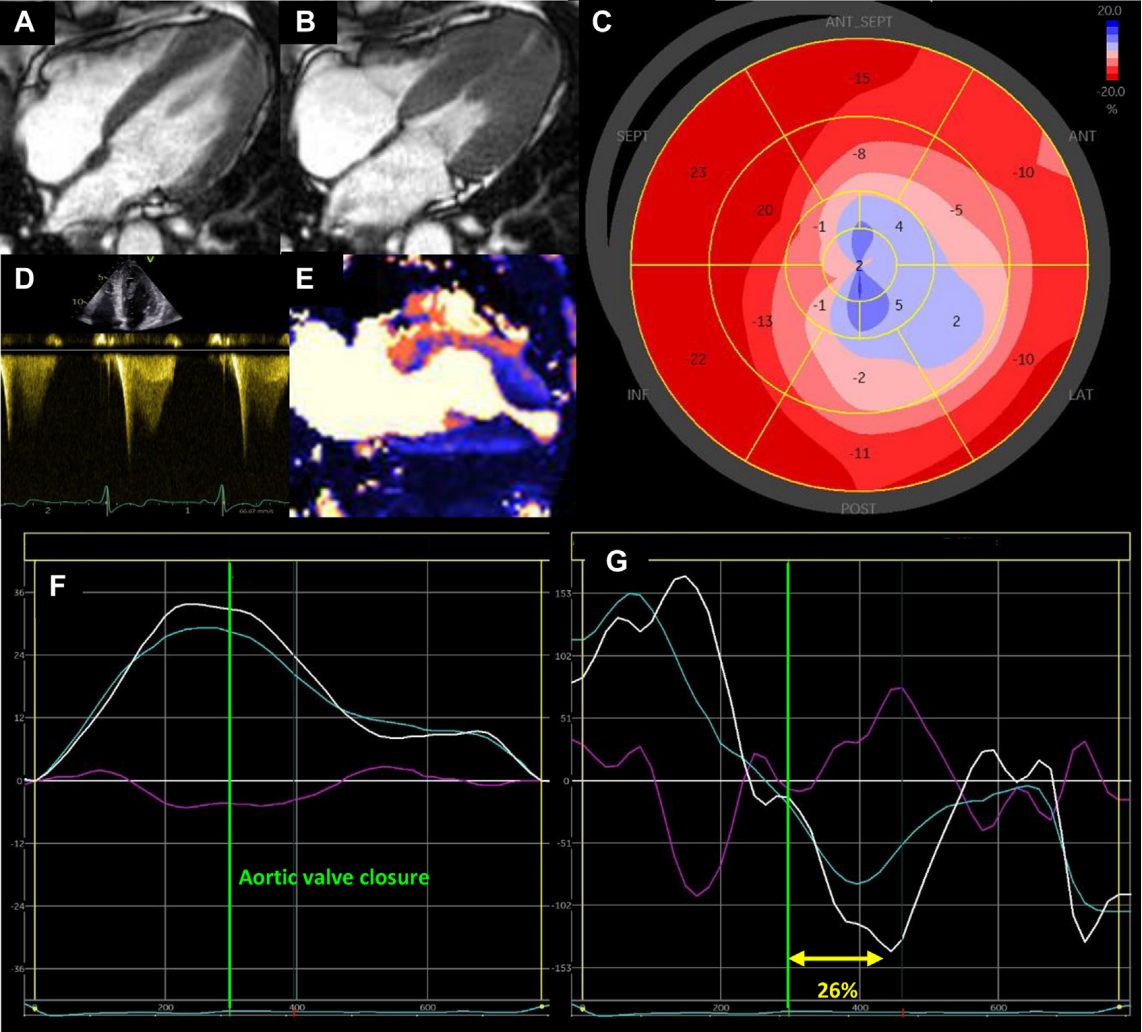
Multiparametric Transthoracic Echocardiographic and Cardiac Magnetic Resonance Imaging Findings in a Patient With Overt Apical Hypertrophic Cardiomyopathy and an Apical Aneurysm (A) CMR 4-chamber image in end-diastole demonstrating apical hypertrophy. (B) CMR 4-chamber image in end-systole showing apical aneurysm with cavity obliteration proximal to aneurysm. (C) Global longitudinal strain bullseye plot showing reduced and paradoxical apical strain typical of an apical aneurysm. (D) Continuous wave Doppler trace through mid-left ventricle on TTE showing classic Doppler appearance of an apical aneurysm with early systolic peak and subsequent signal dropout and a distinct paradoxical diastolic flow jet. (E) 2-chamber stress perfusion CMR map demonstrating impaired apical perfusion. (F) TTE twist graph showing increased apical and LV twist. (G) TTE twist rate graph demonstrating increased rate of apical and LV twist, but delayed peak untwist rate in early diastole at 26%. TTE = transthoracic echocardiography.
